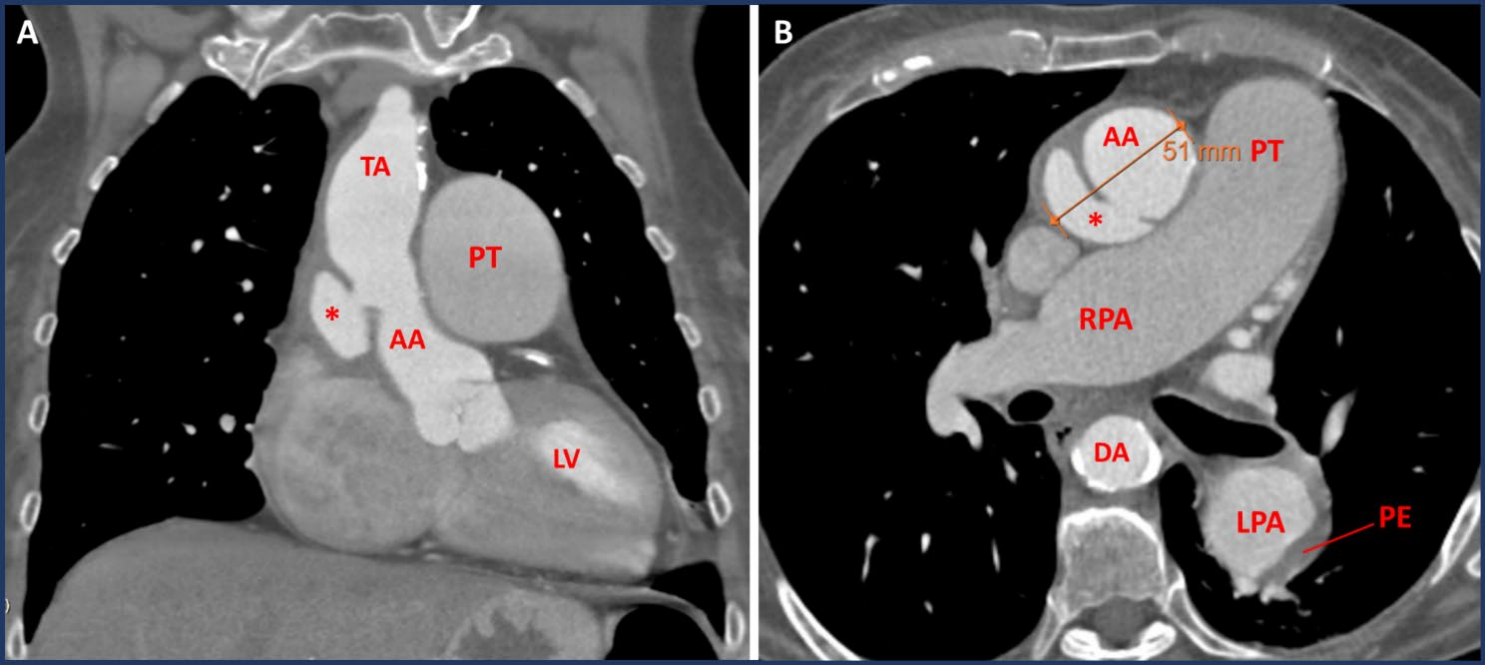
Additional CT angiography of the post-dissection aneurysm. A CT angiography scan of the ascending thoracic aorta, showing the saccular post-dissection aneurysm (depicted by *) at the right-sided posterior section of the mid-ascending aorta in coronal (Fig. 2A) and transversal (Fig. 2B) view AA = ascending aorta; DA = descending aorta; LPA = left pulmonary artery; LV = left ventricle; PE = pulmonary embolism; PT = pulmonary trunk; RPA = right pulmonary artery; TA = transverse aortic arch
